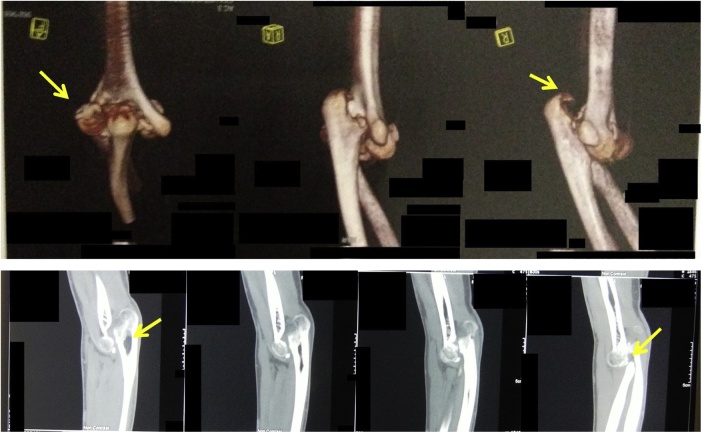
2D and 3D CT Scan reconstruction of the left elbow.
